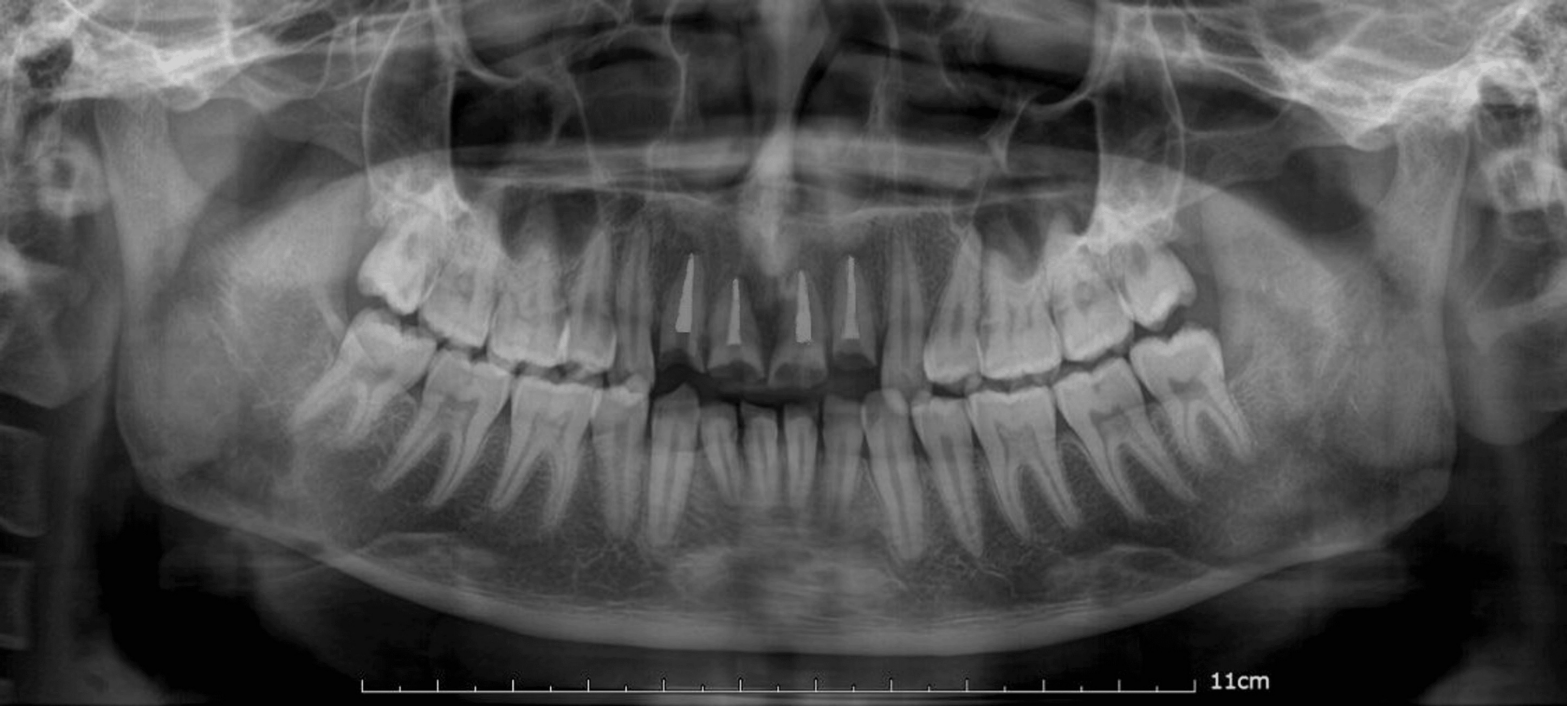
Post-treatment orthopantomogram Root canal treatment #12,11,21 and 22.
